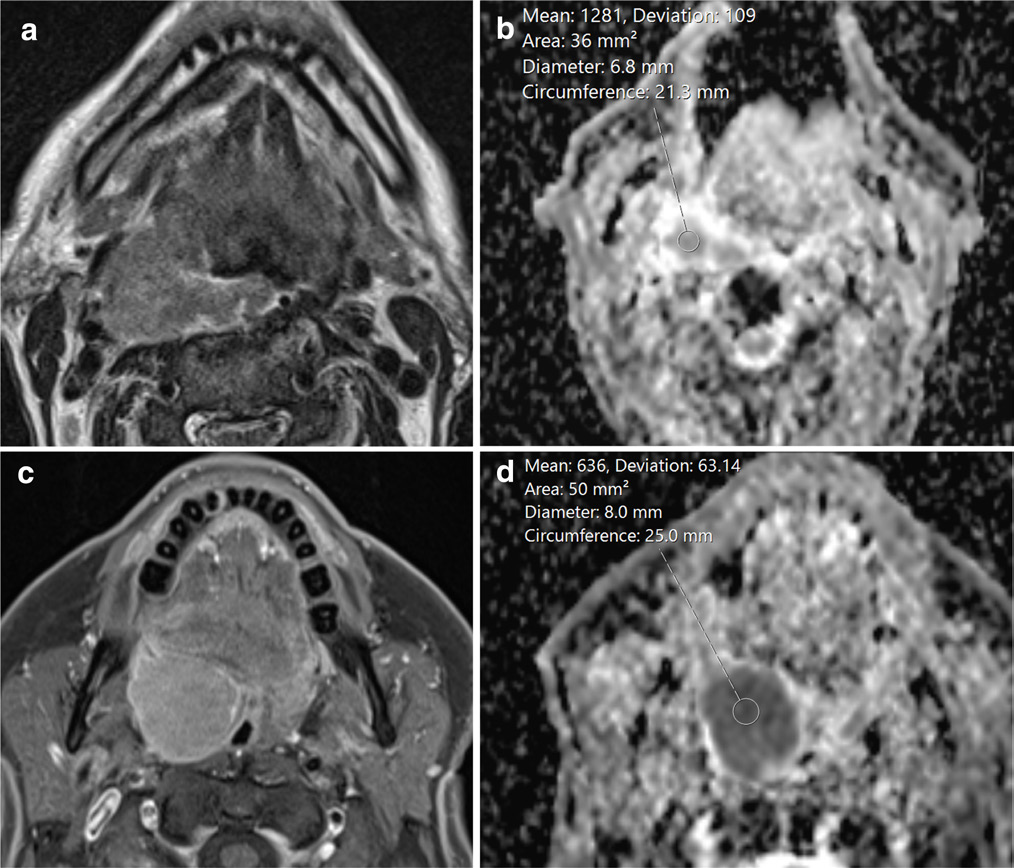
Structural MRI and ADC value differences according to HPV status. Axial *T*
